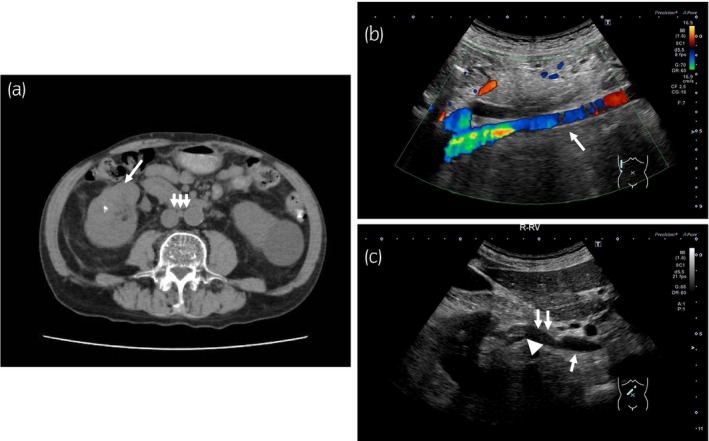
Plain CT image and ultrasonography images after 12 cycles of Nivo‐Cabo. (a) A plain CT image. (b, c) Ultrasonography images. (a) All lesions had regressed 24 weeks after the start day of Nivo‐Cabo. Though the renal mass (single arrow) and the interaortocaval lymphadenopathy (triple arrows) remain, the mass on Gerota's fascia and the tumor thrombus had regressed sufficiently so as not to show on plain CT (b, c) The tumor thrombus did not exist in the IVC (single arrow) given the normal blood flow signal. However, the tumor thrombus (arrowhead) remained within the renal vein (double arrow).
